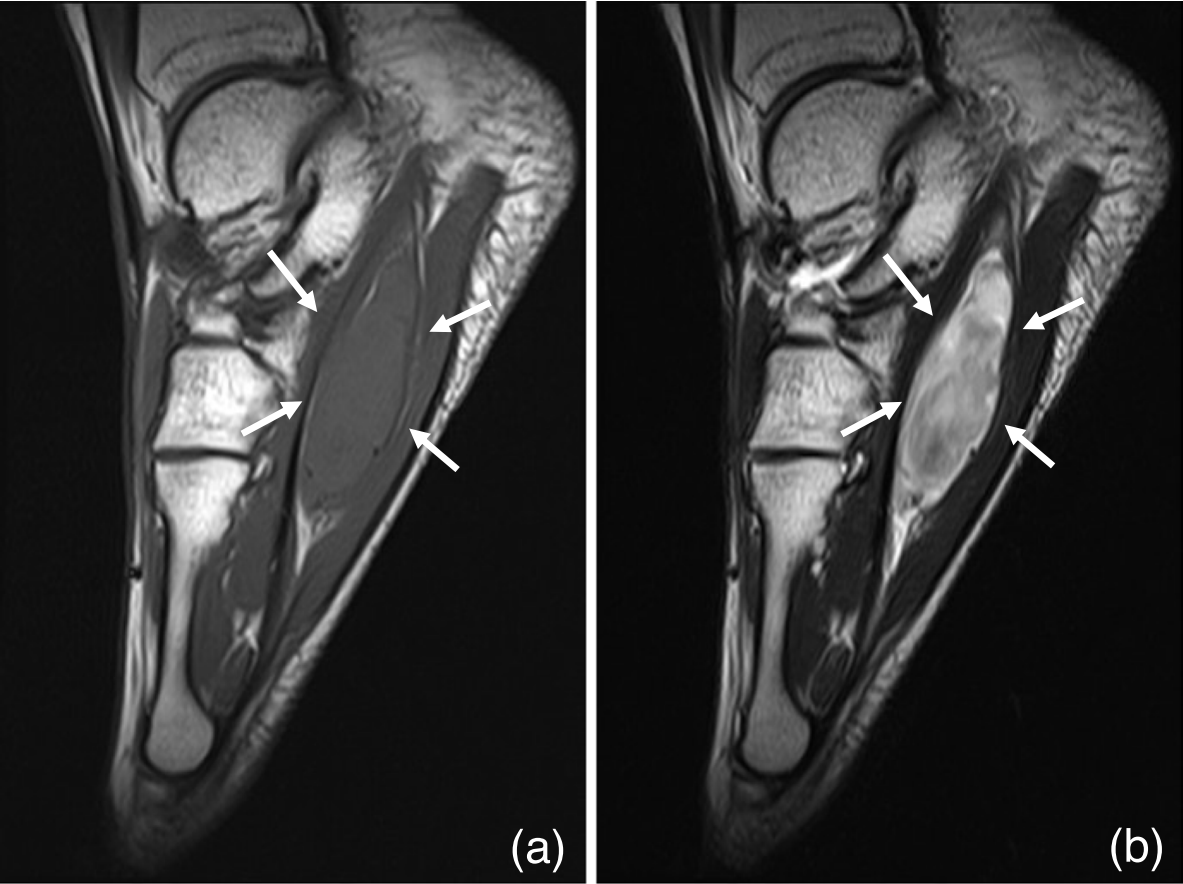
Synovial sarcoma occurred on planta. Sagittal T1 MRI image (a) and T2 MRI image (b) showing plantar lesion, presenting as synovial sarcoma. MRI, Magnetic resonance imaging
